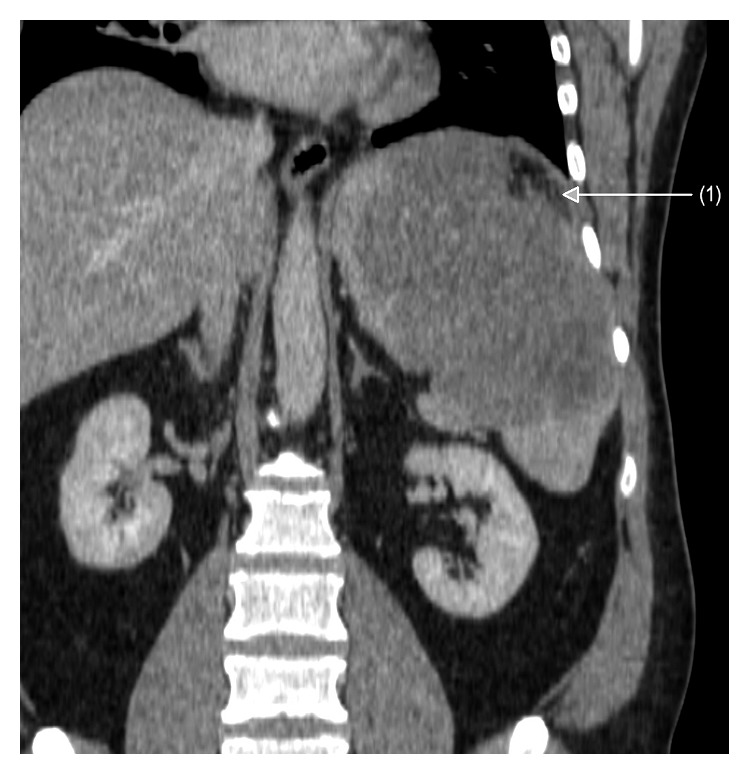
Abdominal CT scan showing an enlarged spleen with its visible rupture (1) and bleeding outside the capsule.
